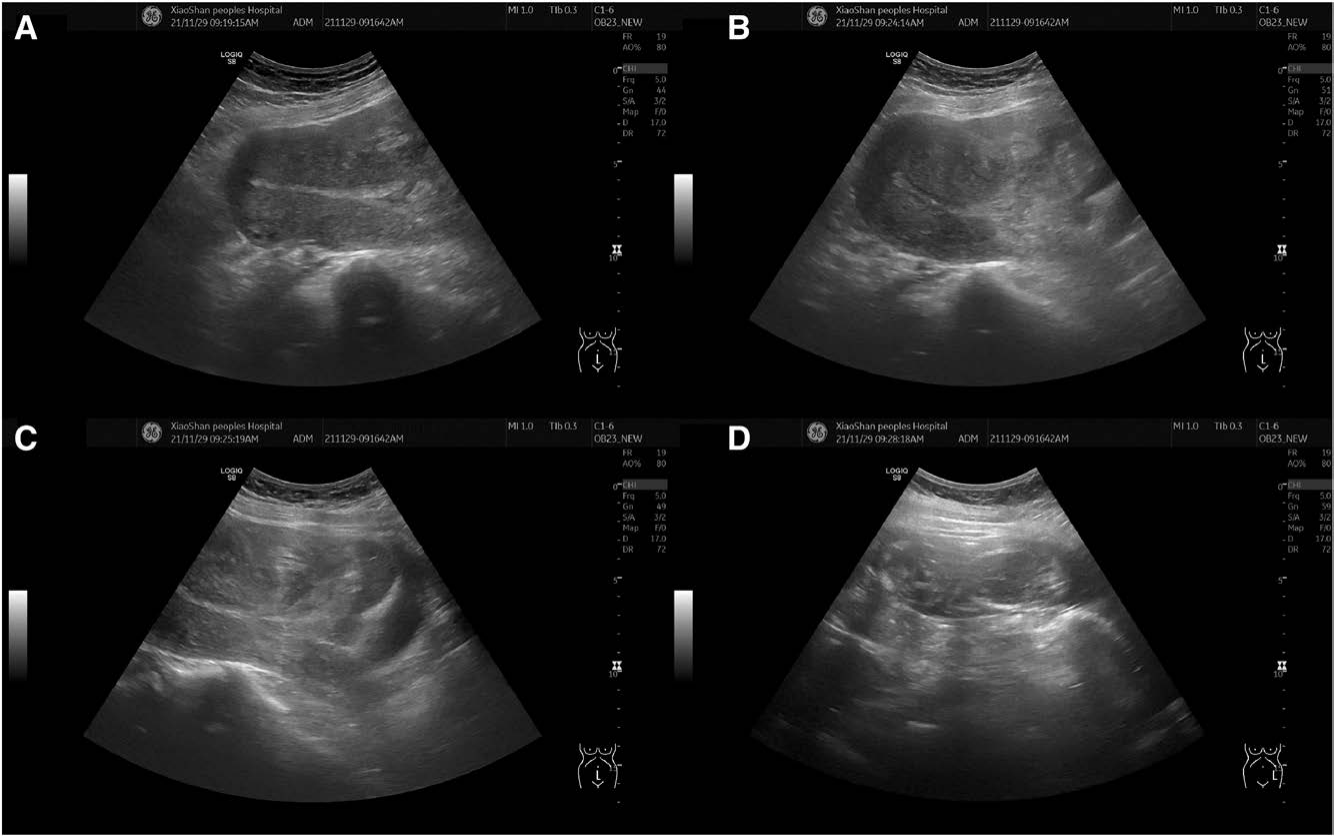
Ultrasonography of uterine rupture during induction of labor in a pregnant woman at 15 + 2 weeks of gestation. A. No visible fetal echoes in the uterine cavity. B. Disruption of myometrial continuity in the lower segment of the anterior wall. C. Mixed clot of placenta seen protruding from the uterine cavity into the abdominal cavity at the incision in the lower part of the anterior wall. D. An amniotic sac-encapsulated fetal echo is seen in the abdominal cavity.
